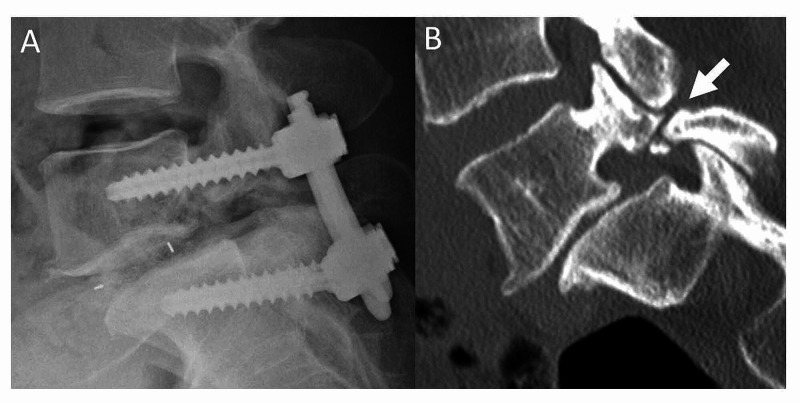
Postoperative radiograph and CT scan A: Post-operative lateral radiograph of a pseudoarthrotic spondylolysis patient who received transforaminal lumbar interbody fusion (TLIF). B: A reconstructed sagittal computed tomography (CT) scan of the same patient taken preoperatively. Arrow indicates a defect of the pars interarticularis. This patient has chronic spondylolysis, which is considered to be pseudoarthrosis.
